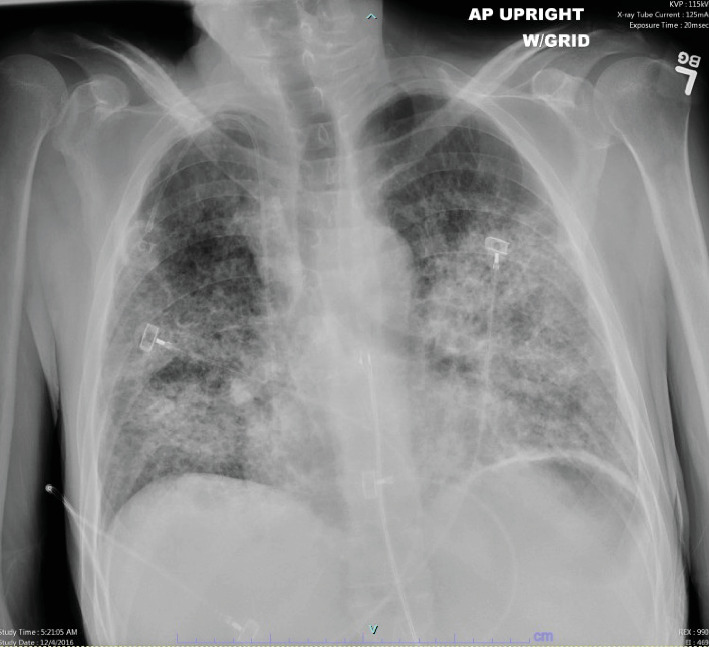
Chest radiograph on readmission to hospital showing stable diffuse interstitial and alveolar opacities predominantly in the mid-lower lungs.
